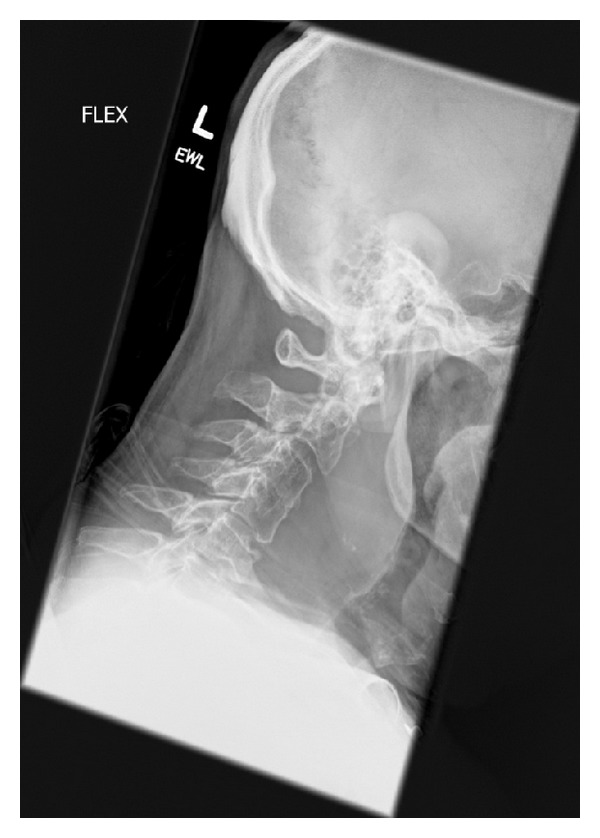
Lateral plain film taking in flexion demonstrating significant prevertebral soft tissue swelling with anterior tracheal displacement.
